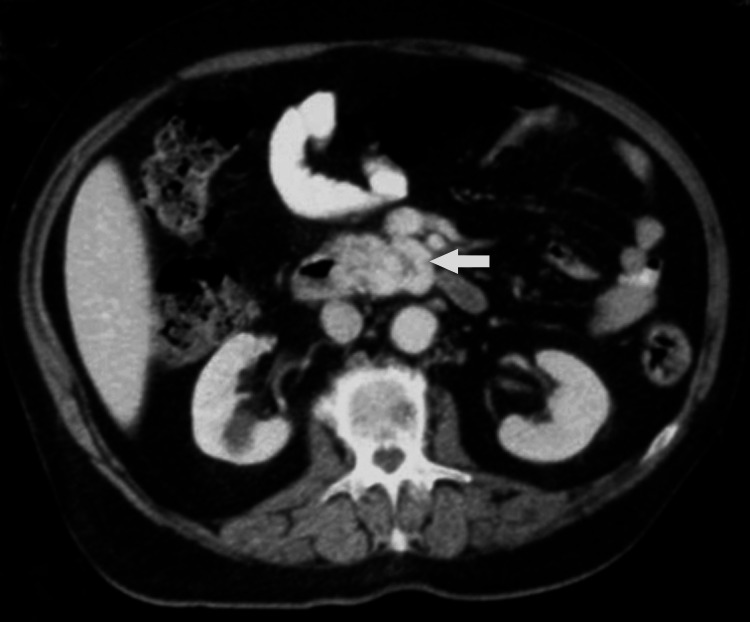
Contrast-enhanced computed tomography of the abdomen. Showing a prominent draining vein coursing towards the superior mesenteric vein (arrow).
